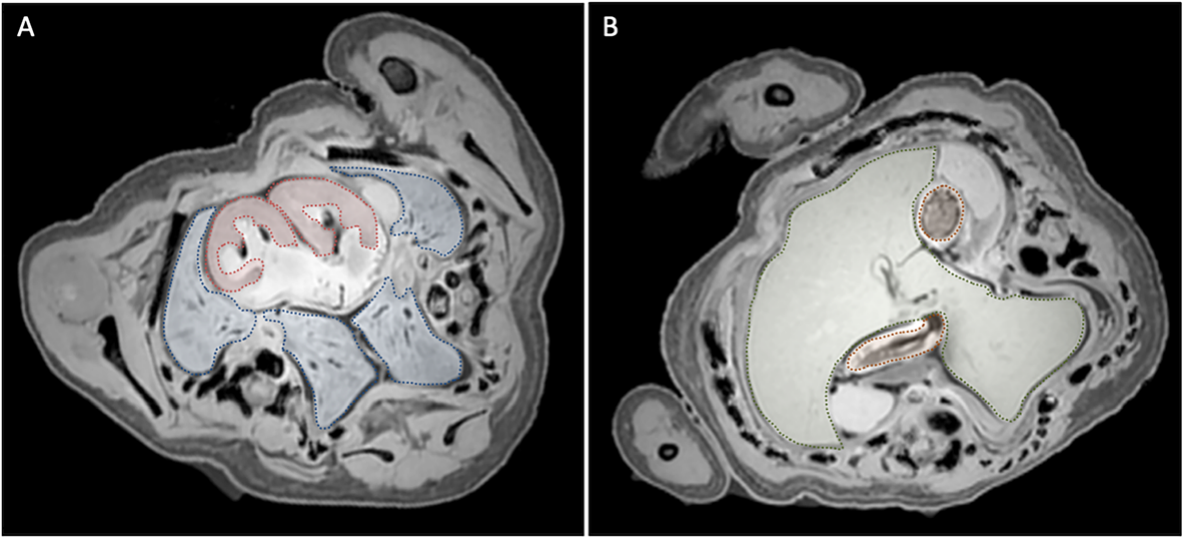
a Axial T1-weighted magnetic resonance (MR) image showing a single atrium with two separate ventricles (*red dotted line*) connected and two pairs of lungs (*blue dotted line*). b Axial T1-weighted MR image showing a single, fused liver (*green dotted line*) and two stomachs (*brown dotted line*)
